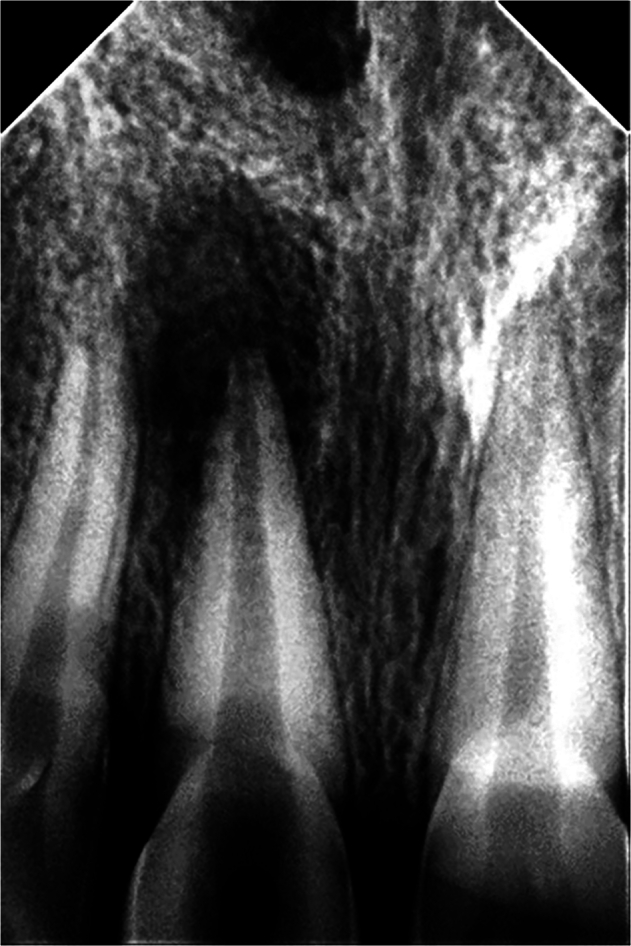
A diagnostic periapical radiograph of large periapical lesion on the maxillary central incisor.
